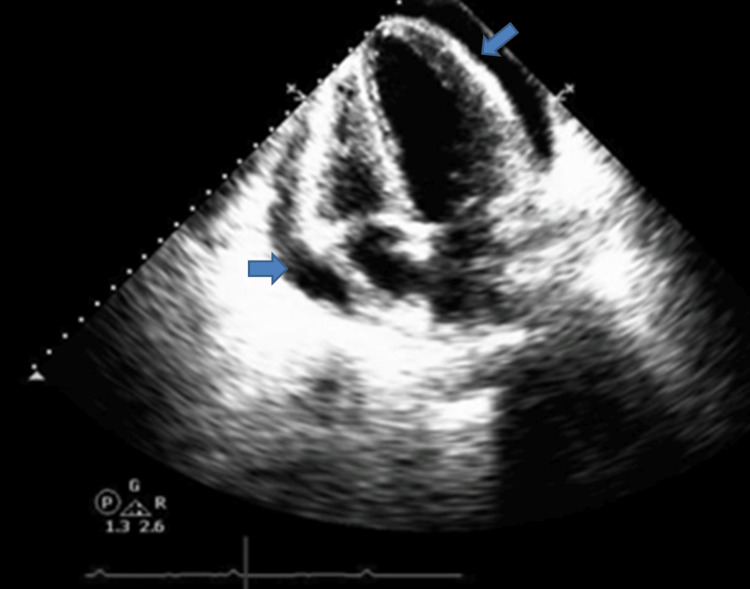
A transthoracic echocardiogram in the apical four-chamber view shows rapid enlargement of a large circumferential pericardial effusion (blue arrow) with right atrial free wall buckling and right ventricular systolic obliteration.
